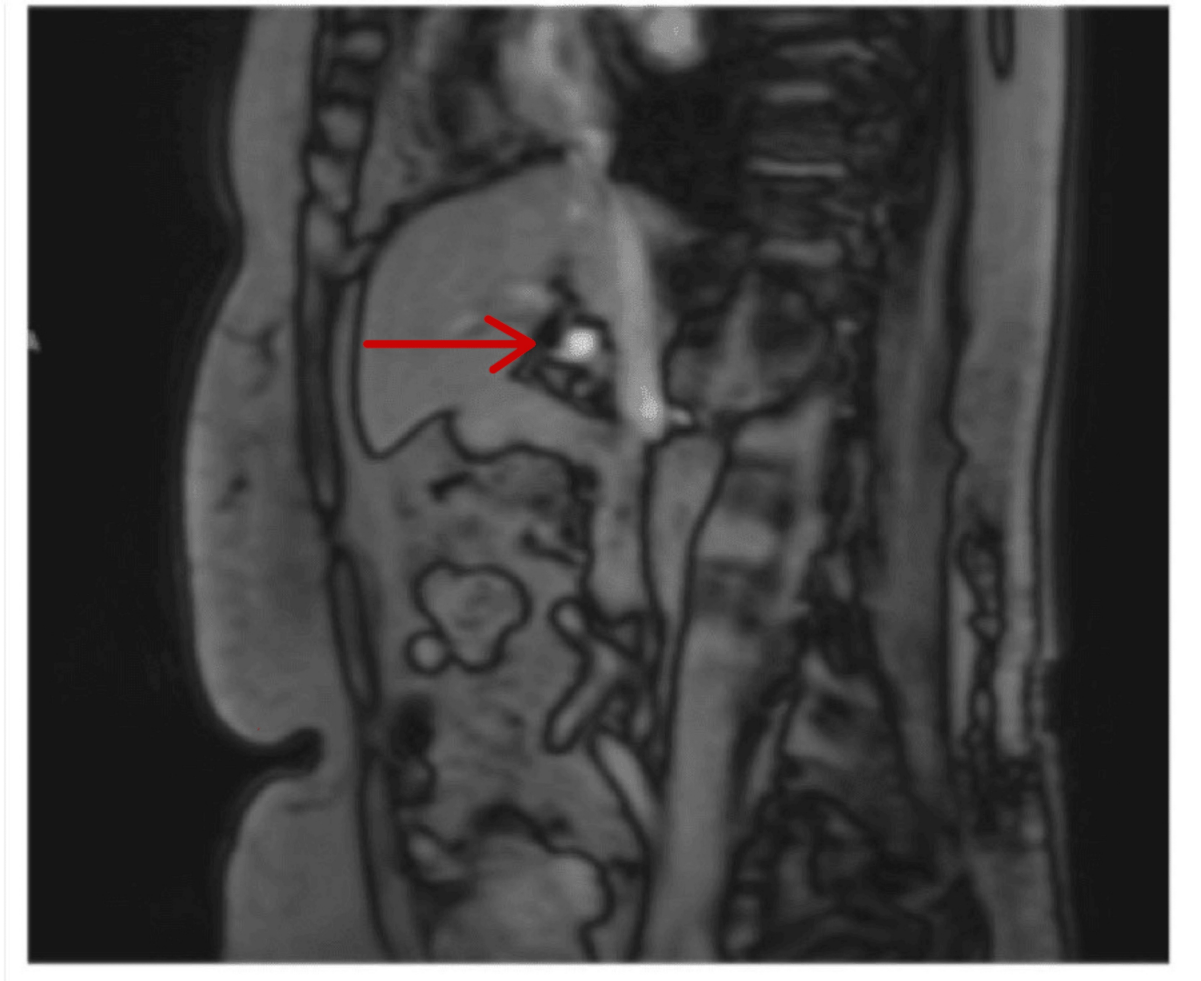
MRI scan showing lesion on pancreatic tail
